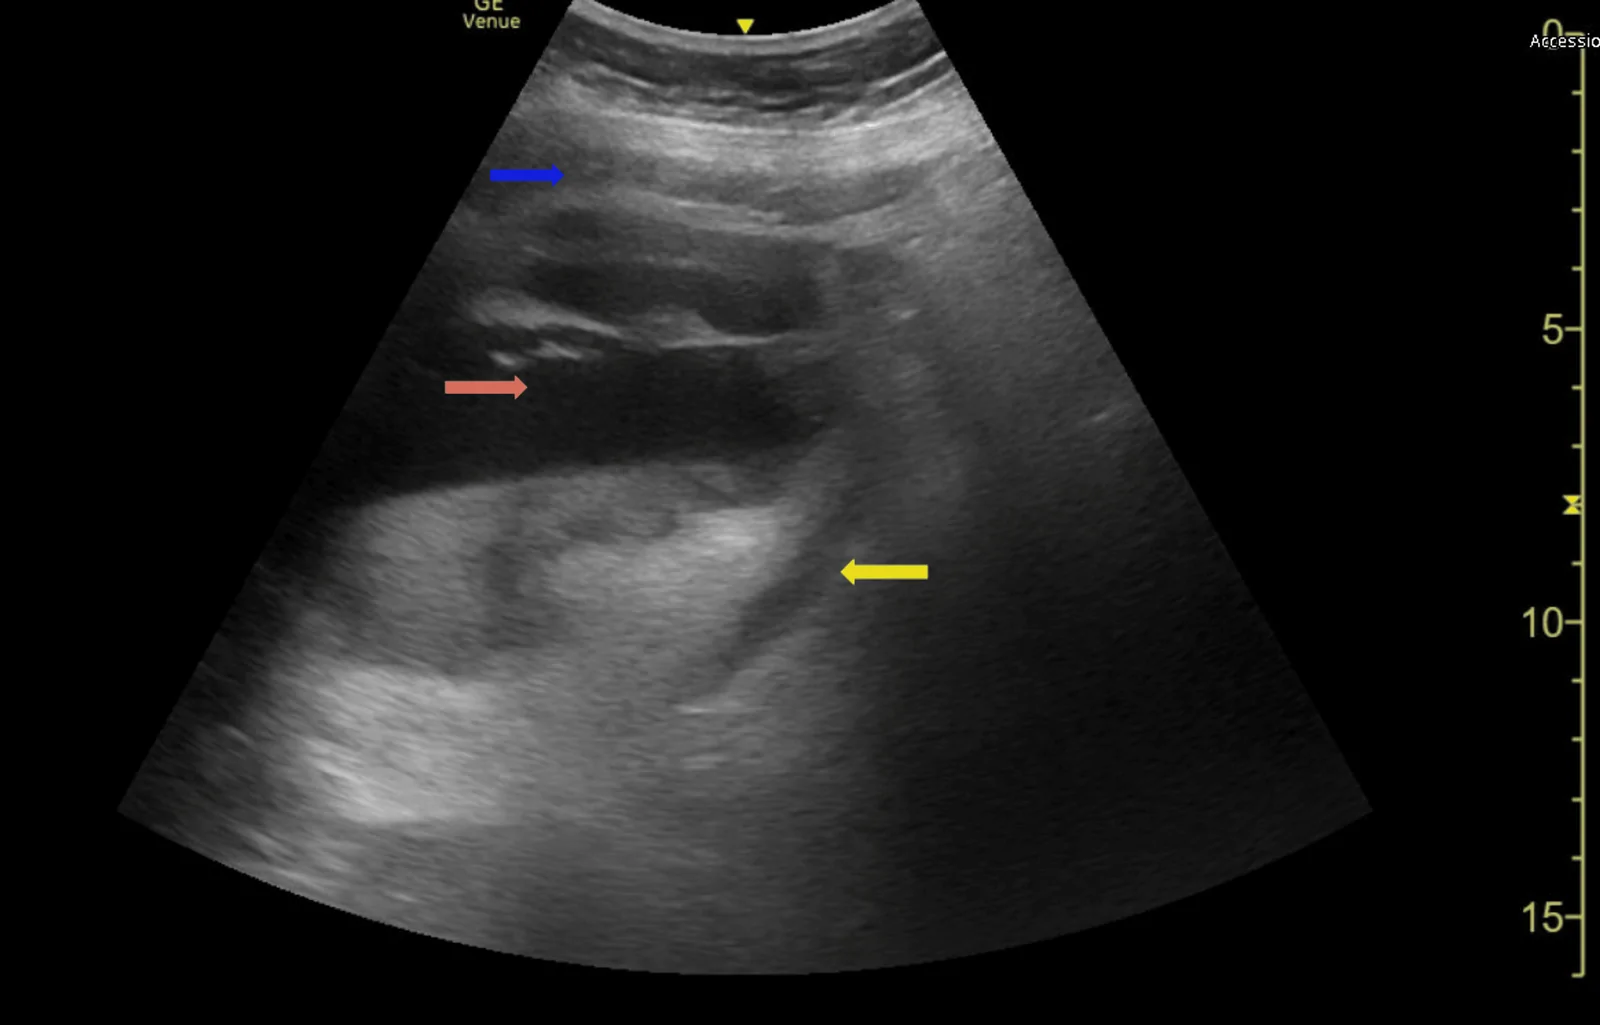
Abdominal POCUS demonstrating a subcapsular hepatic hematoma (blue arrow) and an intraparenchymal hepatic hematoma (red arrow), with free intraperitoneal fluid along the inferior border of the liver (yellow arrow). POCUS: point-of-care ultrasonography
